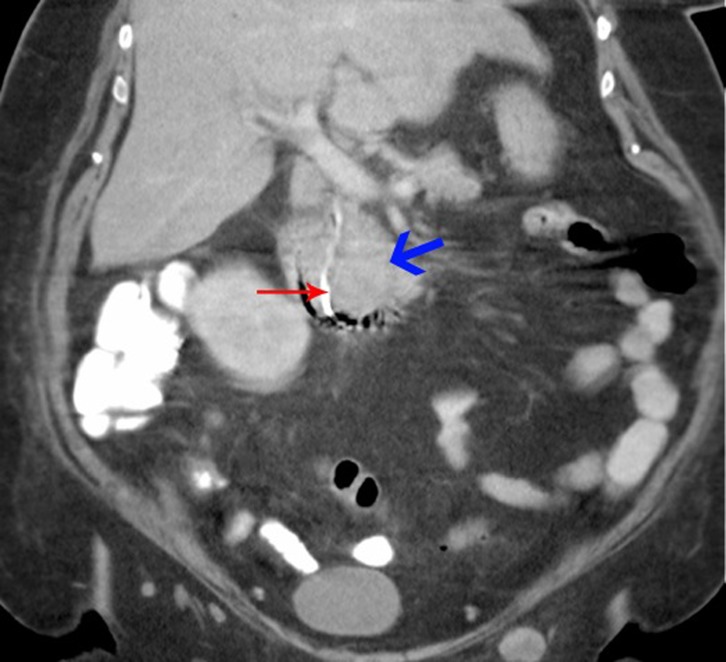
Coronal view of CT abdomen: Blue arrow - tumor deposit; red arrow - biliary stent leading into duodenum.
